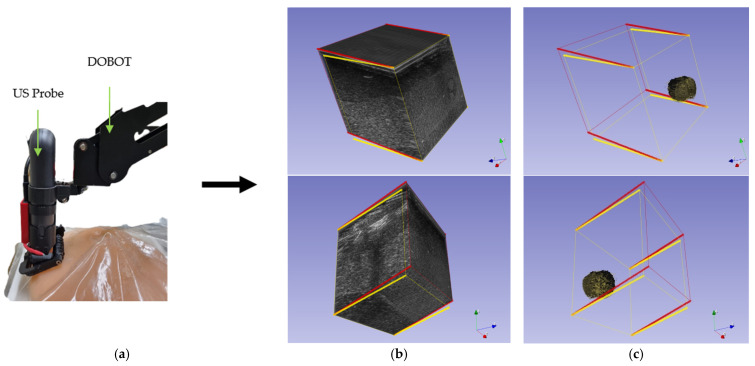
Comparison between the reconstructed volumetric results obtained using the proposed encoder-based freehand ultrasound framework and the Dobot ground-truth reference. The reconstructed 3D ultrasound volume and segmented spherical inclusion are visualized together. The red color indicates the Dobot reference geometry, while the yellow contour represents the proposed reconstruction result. (a) Probe Placement with DOBOT, (b) The reconstructed volumetric results, (c) The segmented spherical inclusions.
